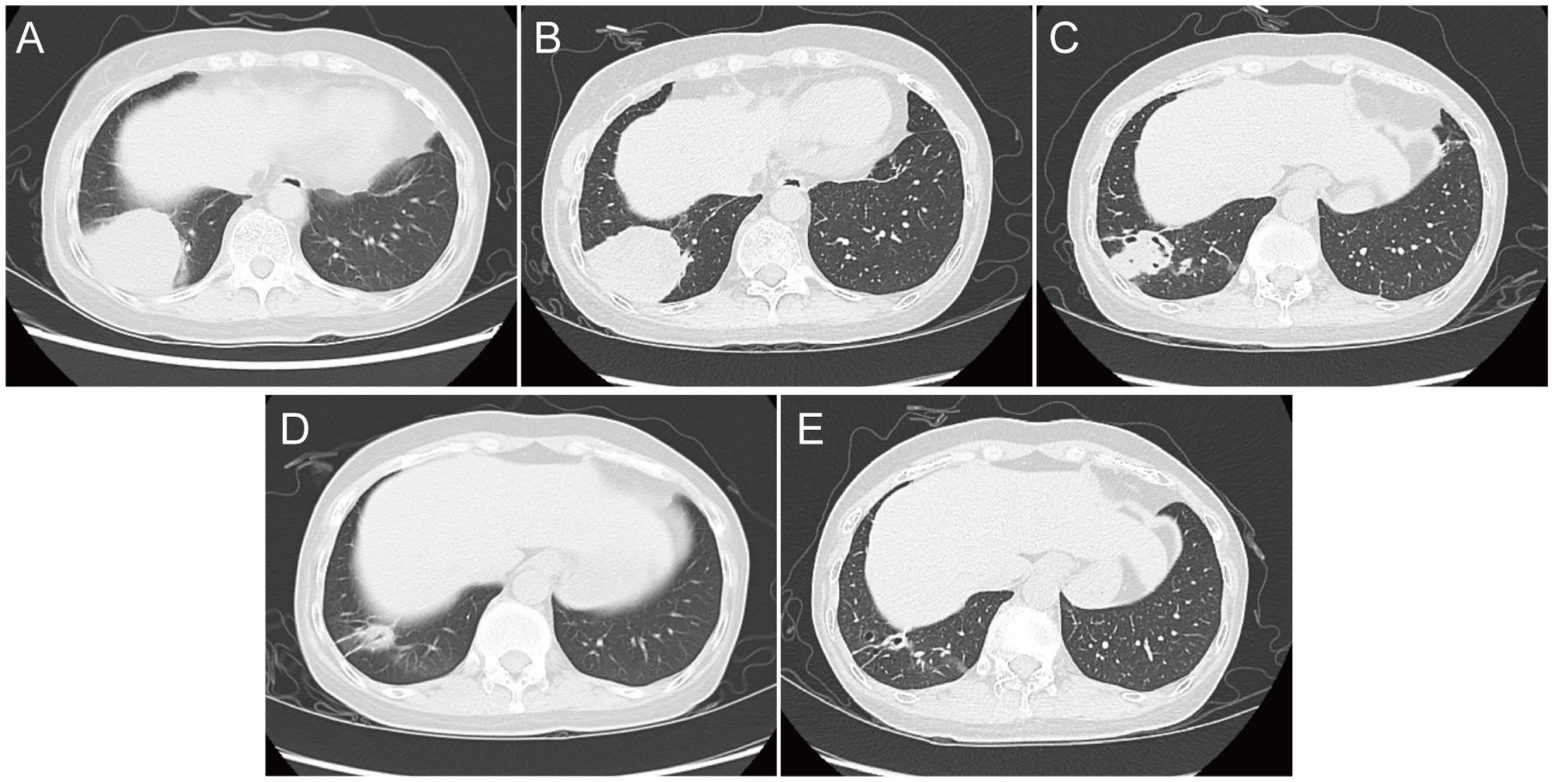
Chest computed tomography image. (A) At admission; (B) on Day 7; (C) on Day 18; (D) at follow-up 1 month after discharge; and (E) at follow-up 4 months after discharge.
